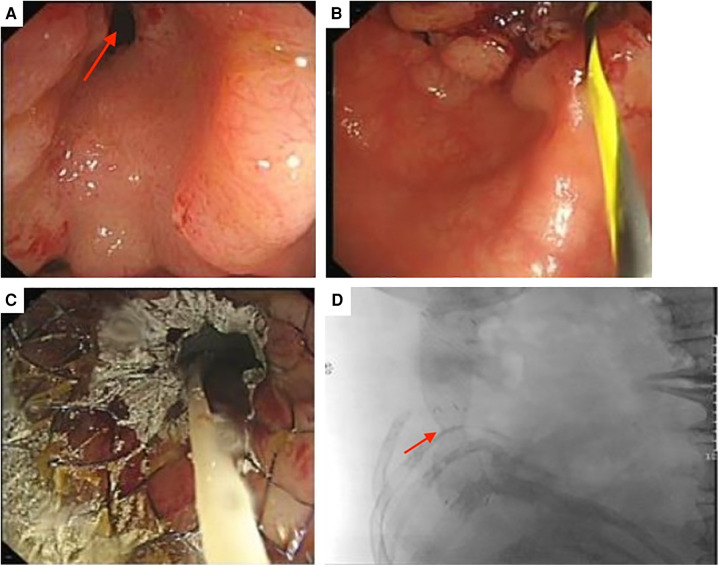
(A) Endoscopy revealing a narrowed intestinal lumen (red arrow). (B) Prior to placement of SEMS (C) successful stent placement relieving intestinal obstruction (D) x-ray showing location of stent and significant relief of intestinal obstruction.
